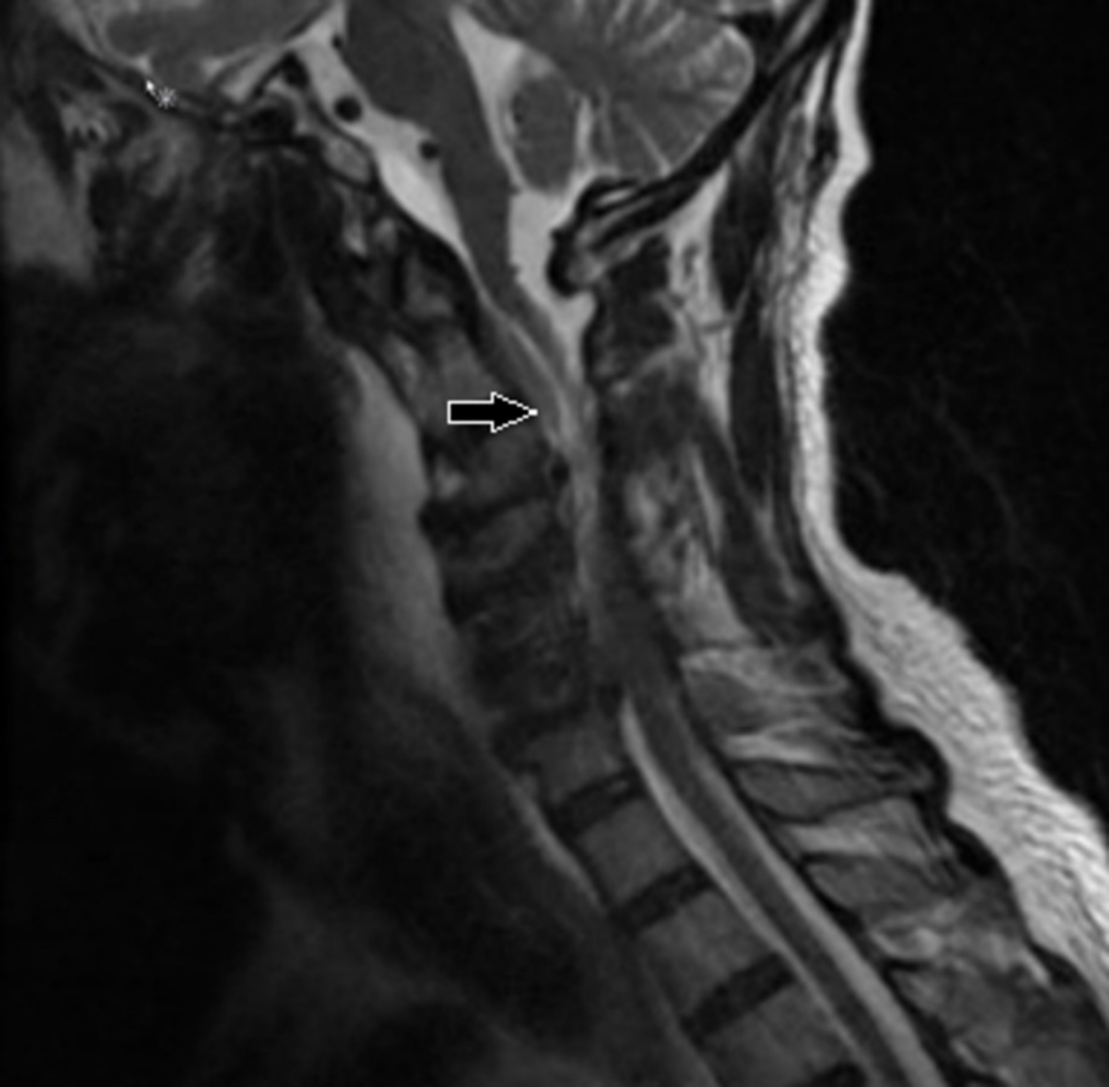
T2-weighted cervical spine MRI in the sagittal plane showing an anterior epidural collection spanning from C2 to C7 with associated mass effect on the spinal cord (arrow)
